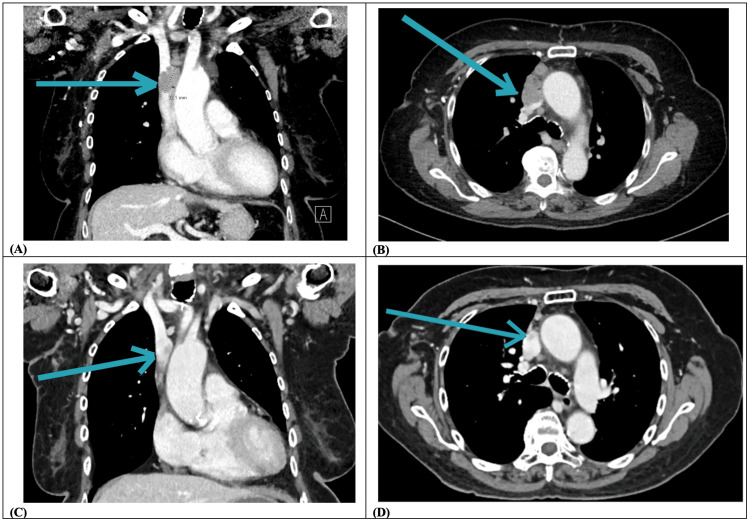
CT images before versus after EBRT. (A) June 2024: Coronal view of CT neck and chest with contrast showing 2/3 luminal occlusion of the SVC (blue arrow). (B) June 2024: Axial view of CT neck and chest with contrast showing the same finding (blue arrow) as in (A). (C) June 2025: Coronal view of CT neck and chest with contrast showing near-complete regression of the tumor thrombus within the SVC (blue arrow). (D) June 2025: Axial view of contrast-enhanced CT of the neck and chest showing the same finding (blue arrow) as in (C). EBRT, external beam radiotherapy
